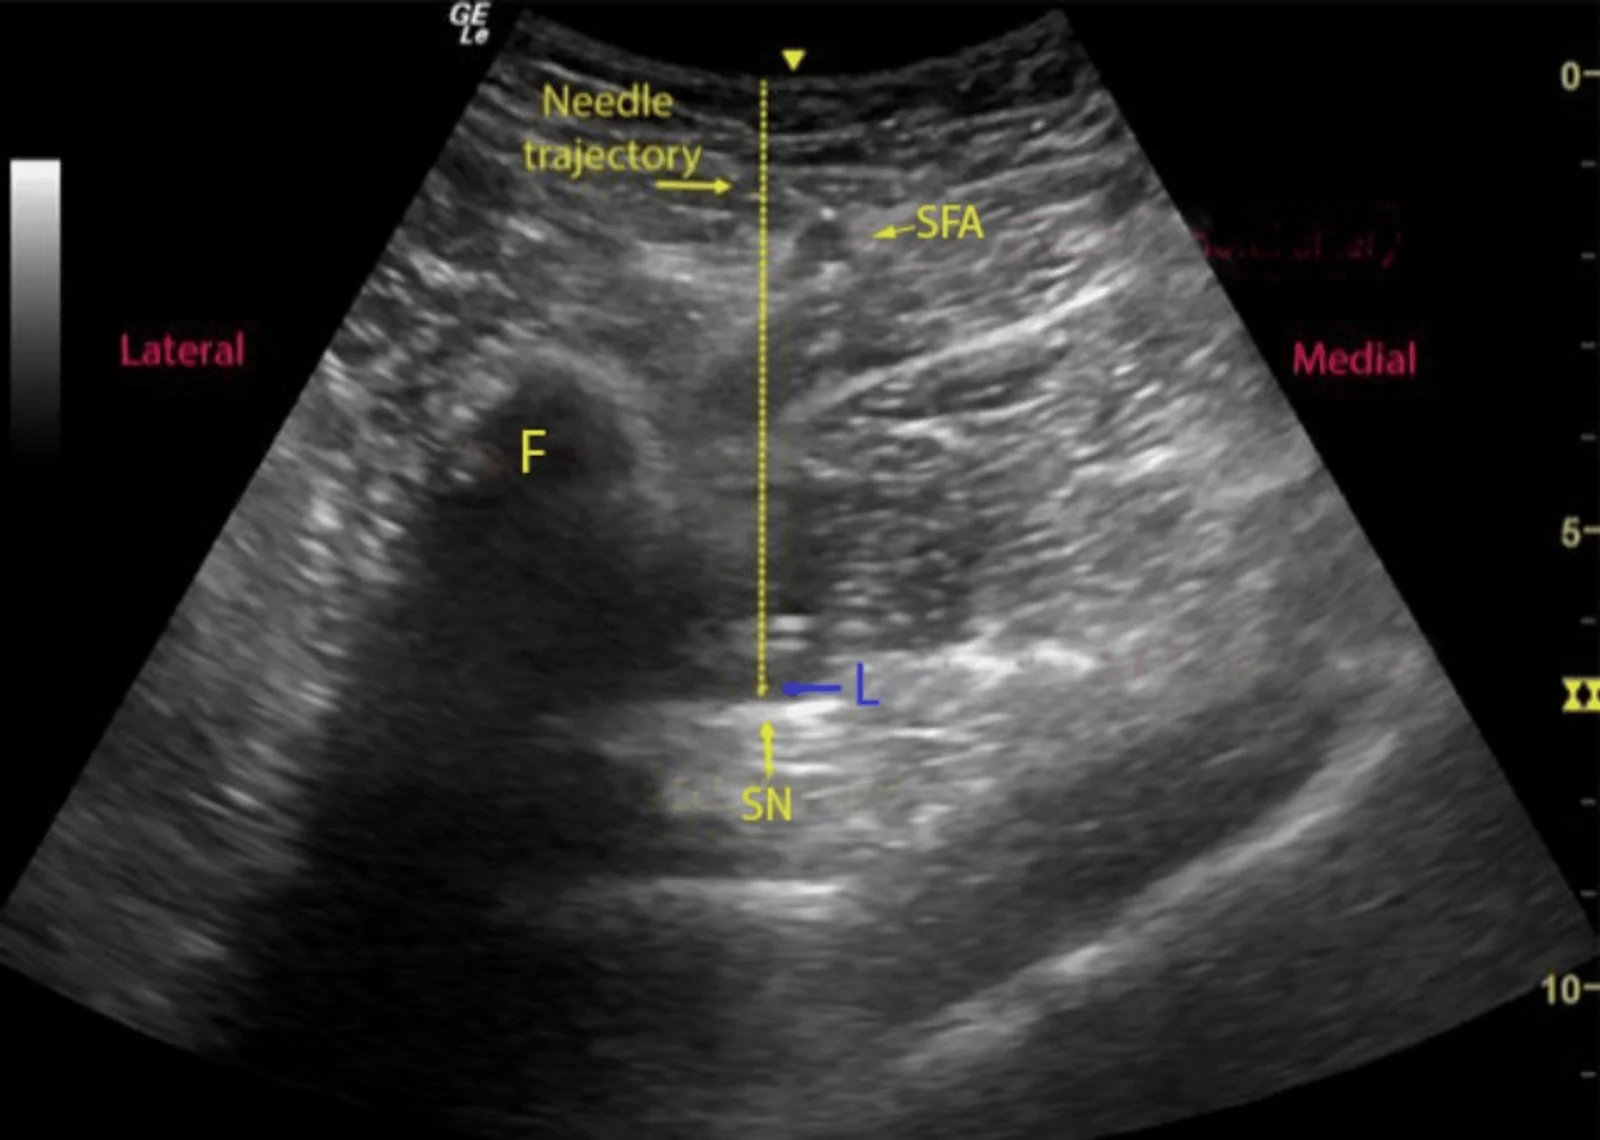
The ultrasound image immediately after local anesthetic injection. Note the local anesthetic (L) inferior to the adductor fascia. The needled trajectory is demonstrated (dashed line) The sciatic nerve (SN) is visualized within the local anesthetic and has moved inferiorly from the adductor fascia. Source: Halpern et al., 2022 [6]; reproduced with permission from Taylor & Francis Informa UK Ltd.
